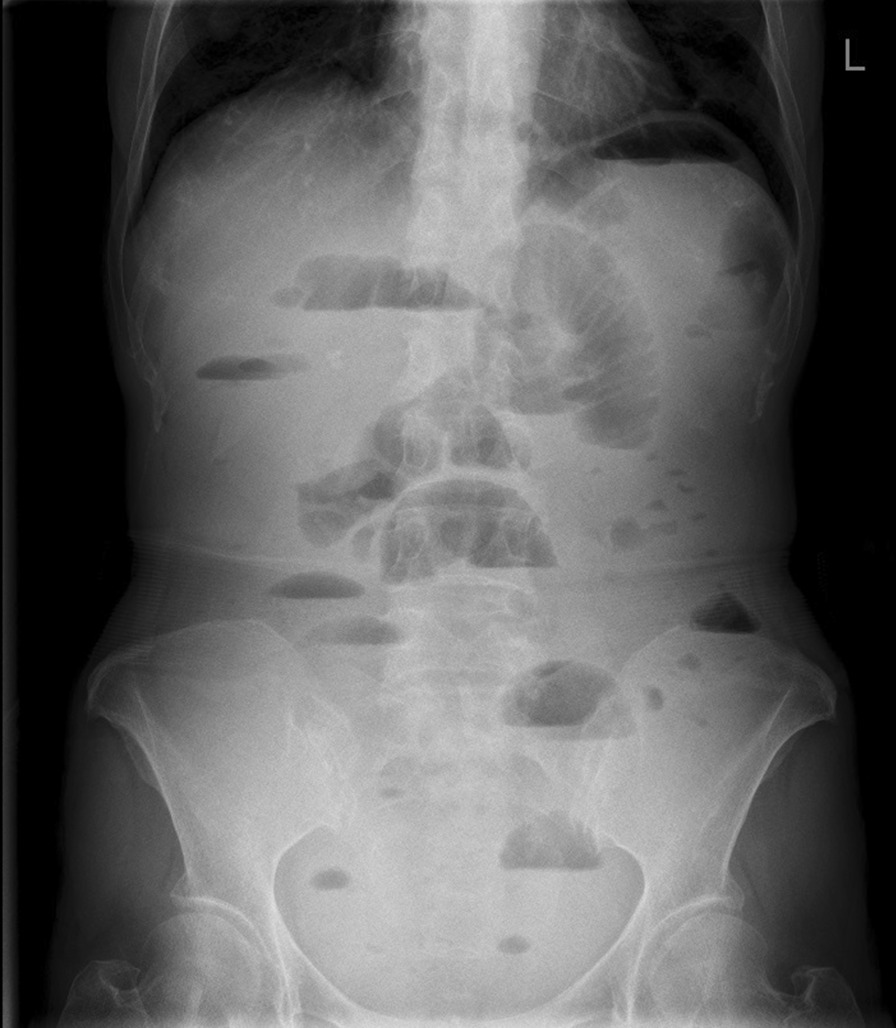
Plain abdominal radiography suggest intestinal obstruction
